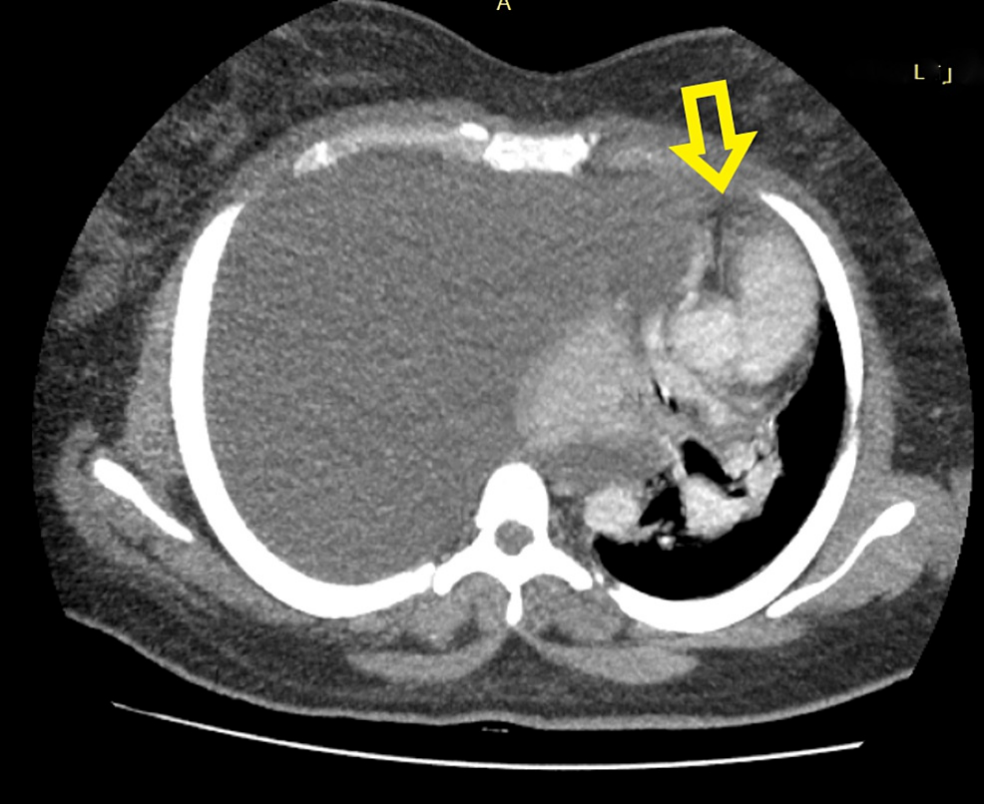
Preoperative CT scan Right hemi-thoracic, pleural-based soft tissue mass with massive pleural effusion causing contralateral mediastinal shift and mass effect on the heart.
